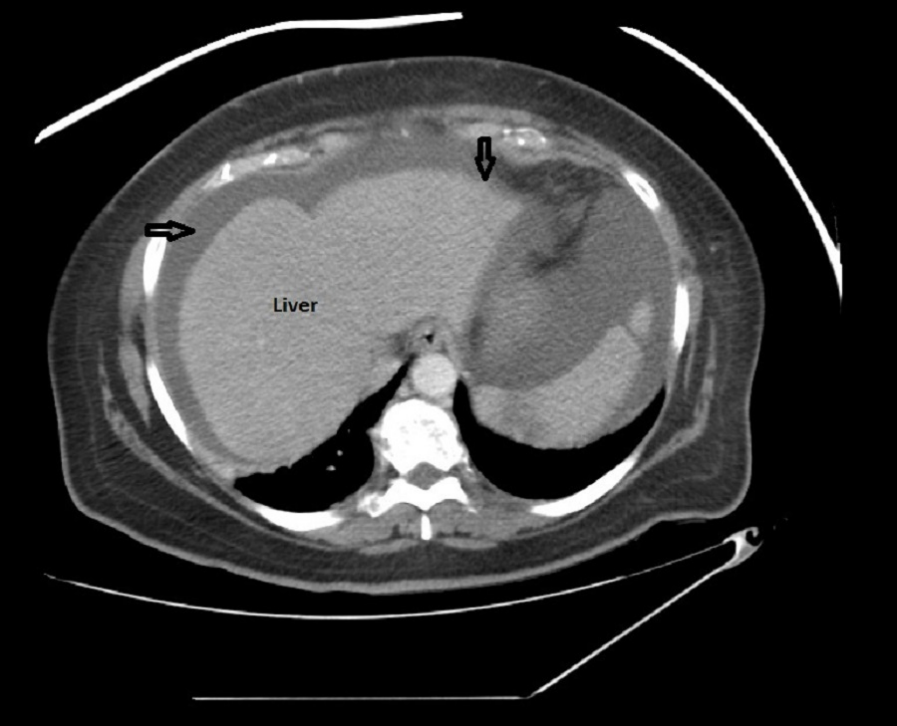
Computed tomography (CT) scan of the abdomen showing a nodular contour of the liver with moderate abdominopelvic ascites.
